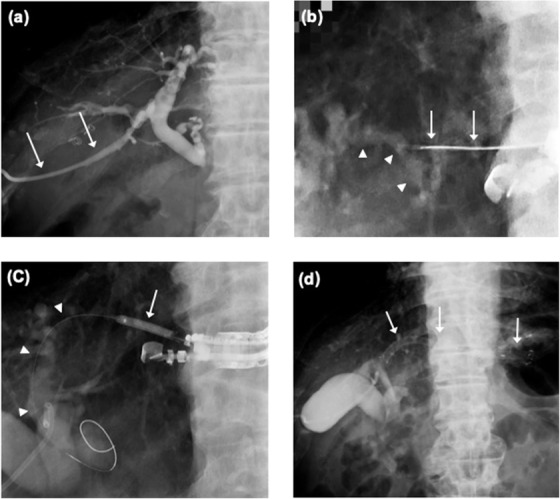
Conversion of PTBD to EUS‐HGS. (a) Cholangiography via the PTBD catheter (arrows). There is no contrast in the distal common bile duct and duodenum due to tumor obstruction of the distal common bile duct. (b) Fluoroscopic image showing the puncture of a 19‐gauge needle (arrows) into the left intrahepatic bile duct (arrow heads). The puncture point can be clearly identified. (c) Fluoroscopic image showing the insertion of a 0.025‐inch guidewire (arrowheads) into the bile duct followed by dilation of the puncture tract with a 4‐mm biliary dilation balloon catheter (arrow). (d) Fluoroscopic image showing placement of a covered metallic stent (arrows) at the appropriate position Abbreviations: EUS‐HGS, endoscopic ultrasound‐guided hepaticogastrostomy; PTBD, percutaneous transhepatic biliary drainage.
